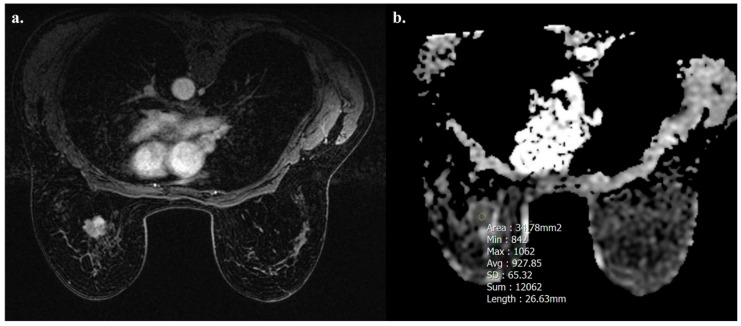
A 52-year-old woman with NST BC subtype Luminal B with low TIL levels (3%) in the left breast. Axial T1-weighted contrast-enhanced MR image shows heterogeneously enhanced mass (a), and hypointensity on ADC map (0.927 × 10^−3^ mm^2^ s^−1^), as showed by the circle (b).
